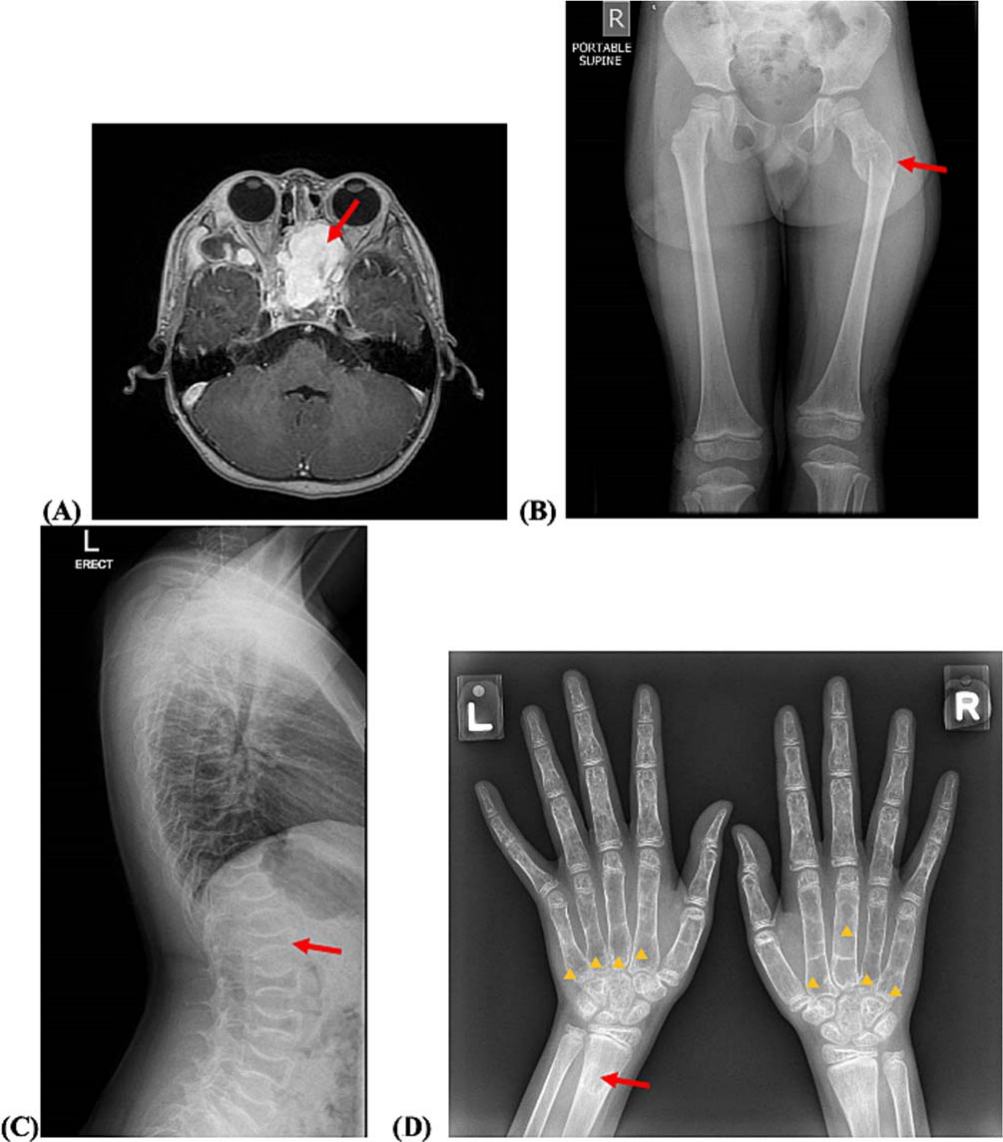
(A) MRI orbits demonstrating left sphenoid vascular malformation (arrow) at initial presentation. (B) Radiograph (antero-posterior view) of bilateral hips demonstrating patient’s first left proximal femur fracture with an underlying expansile lytic lesion (arrow). (C) Radiograph (lateral view) of the spine demonstrating central narrowing of vertebral bodies giving a “fish-like” appearance. (D) Radiograph (antero-posterior view) of bilateral hands demonstrating multiple lucent lesions with cortical irregularity and osteopenia (arrowheads), a lucent lesion in left distal radius with narrow zone of transition (arrow), and a healing fracture over the right distal radius.
